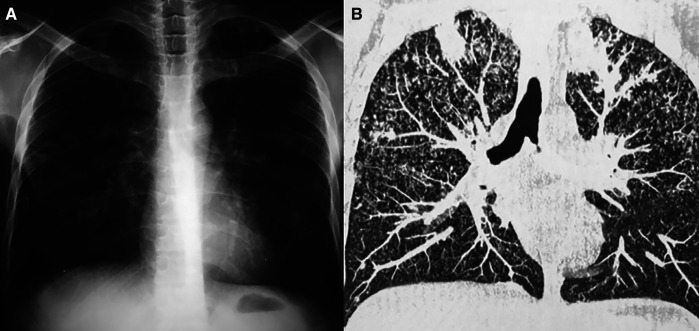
Chest radiograph (A) showed only nonspecific opacities. Computed tomography (B) revealed numerous cavitations along with the classic miliary pattern.
